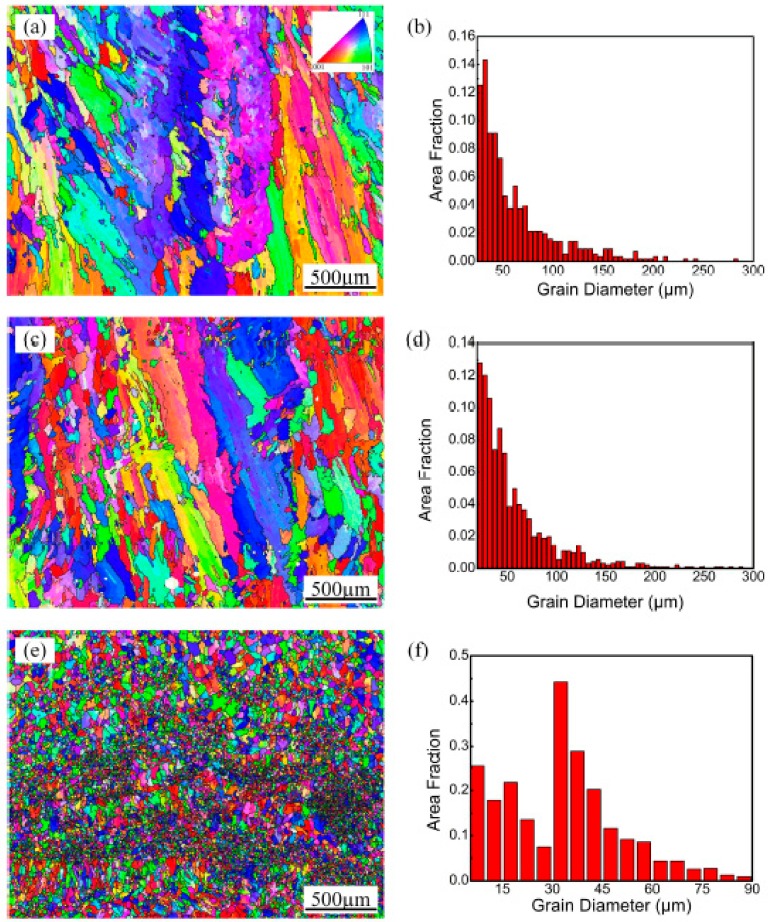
Inverse pole figure (IPF) colored orientation image map and grain size distribution of (a,b) As-prepared sample; (c,d) Remelting treatment sample; (e,f) Remelting treatment sample with ultrasonic vibration.
